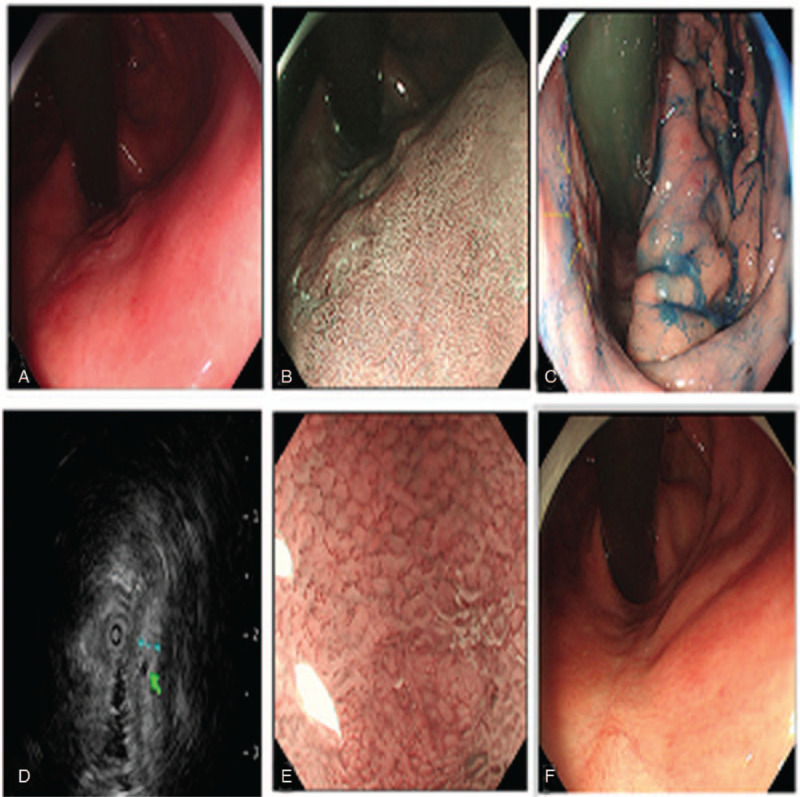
Endoscopic imaging of the lesion in the posterior wall of cardia. White light imaging showed a slightly elevated lesion in the posterior wall of cardia (A). NBI and indigo carmine spraying revealed a well demarcated line around lesion (B and C). EUS revealed a hypoechoic mass located in the first layer and no echo tube structures in the second layer (D). ME-NBI revealed an absent microsurface pattern and a fine network of irregular microvessels on the tumor surface (E). Endoscopic examination 3 months after ESD reviewed a scar (F). ESD = endoscopic submucosal dissection, EUS = endoscopic ultrasonography, ME-NBI = magnifying endoscopy with narrow band imaging, NBI = narrow band imaging.
